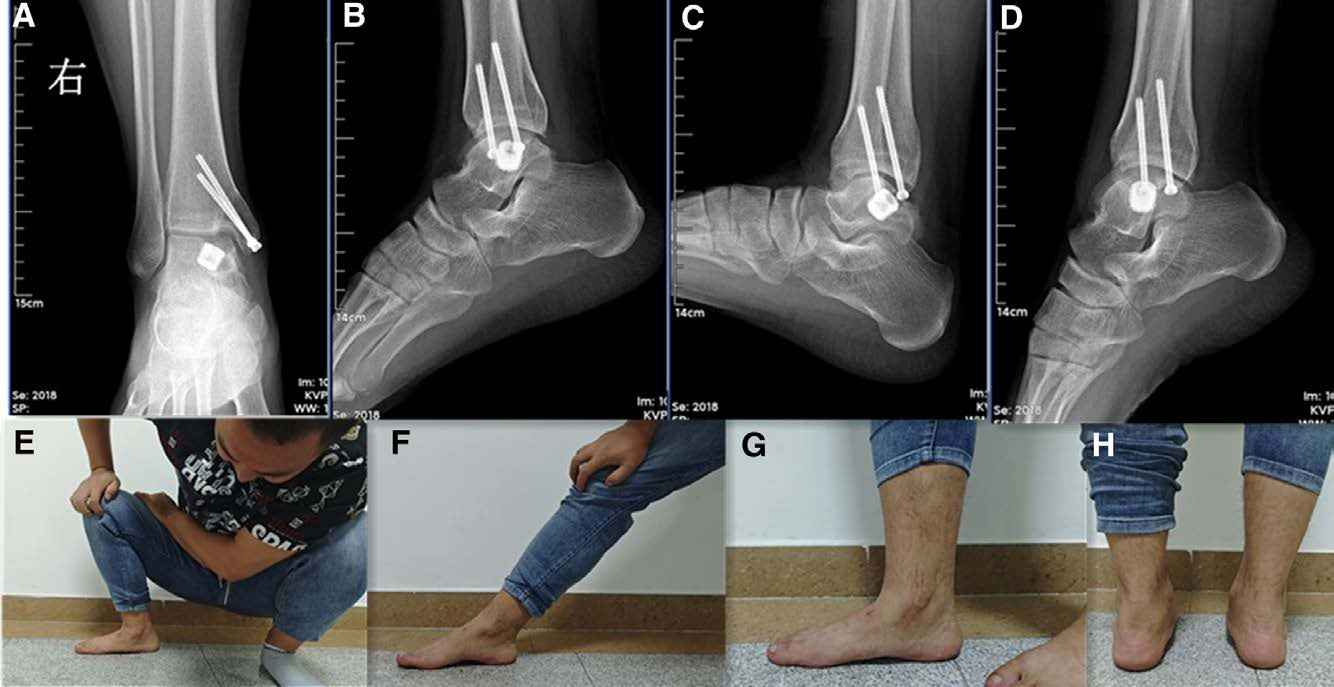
X-rays after partial talar surface replacement after 2 years of surgery (A–D). The function of ankle after 2 years of surgery (E–H).
